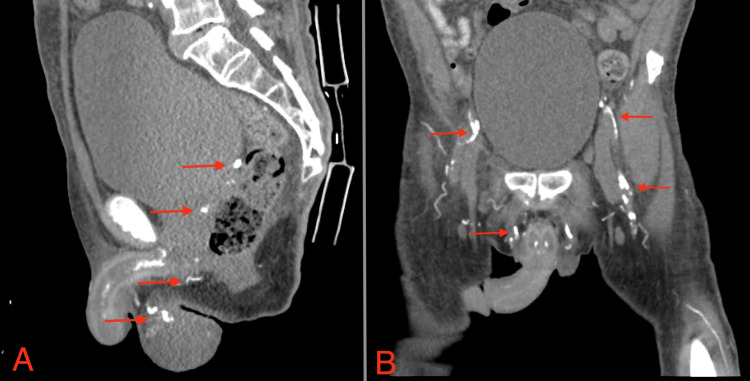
CT of the pelvis without contrast: (A) lateral view; (B) anterior view Arrows depict calcified content
